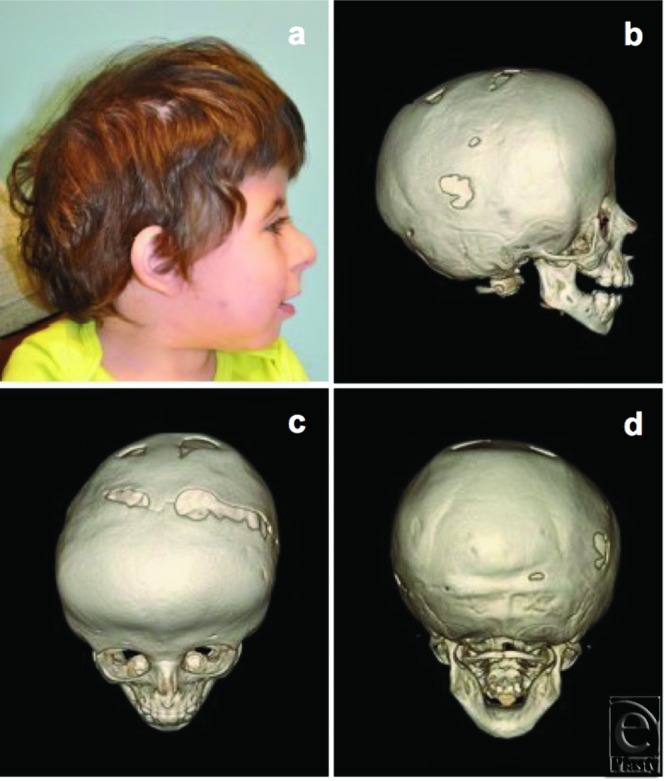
Clinical (*a*) and radiographic appearance (*b-d*) 1 year after posterior vault distraction reveals pan-suture synostosis. Routine computed tomography demonstrated interval closure of the sagittal and lambdoid sutures.
